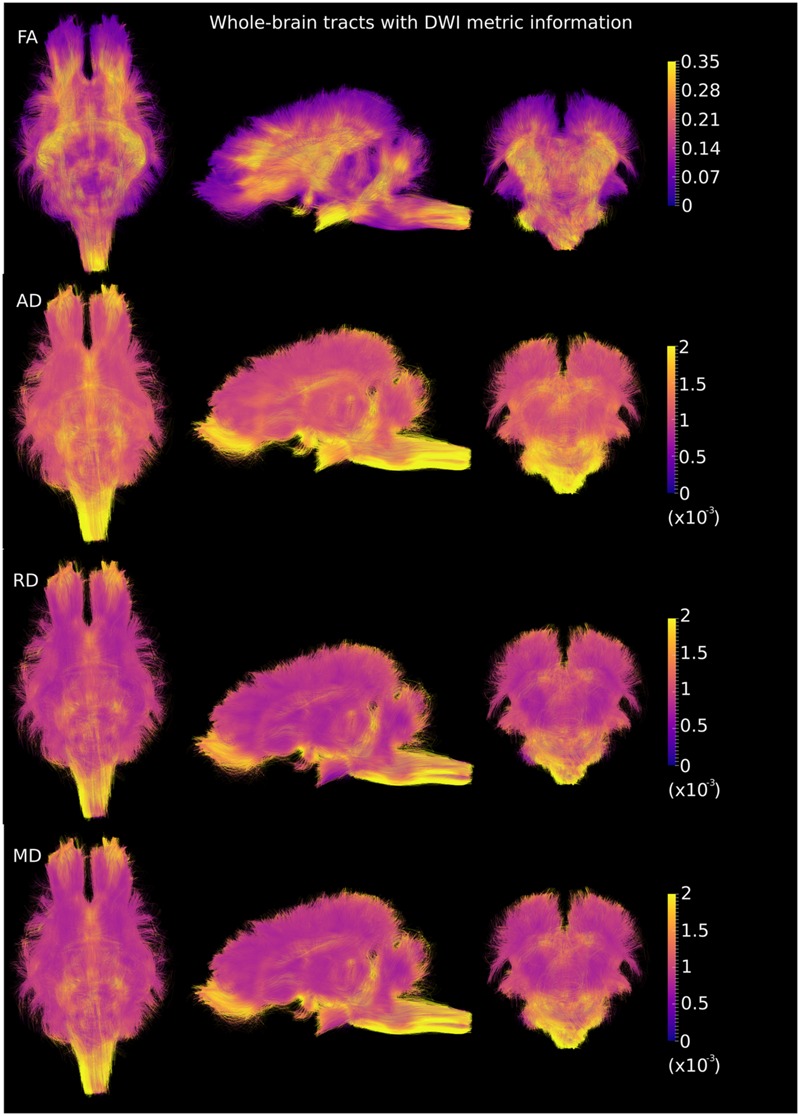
Merged whole-brain fiber tracts overlaid with DWI metrics (with the same value scale as in Figure 2) in the axial, sagittal and coronal views. Brighter color represents higher values.
